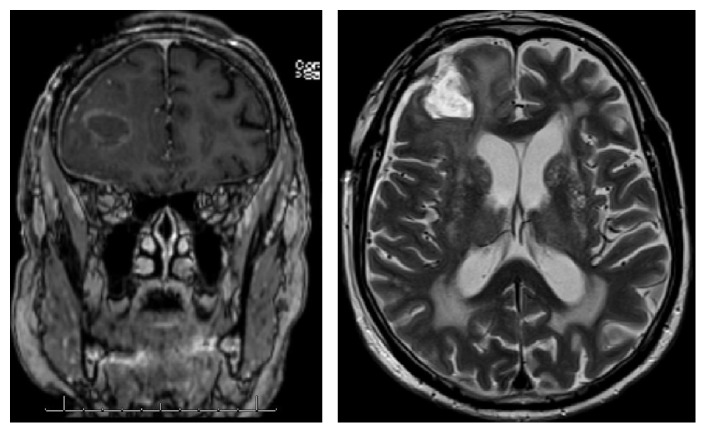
Cerebral MRI: right frontal lesion.
